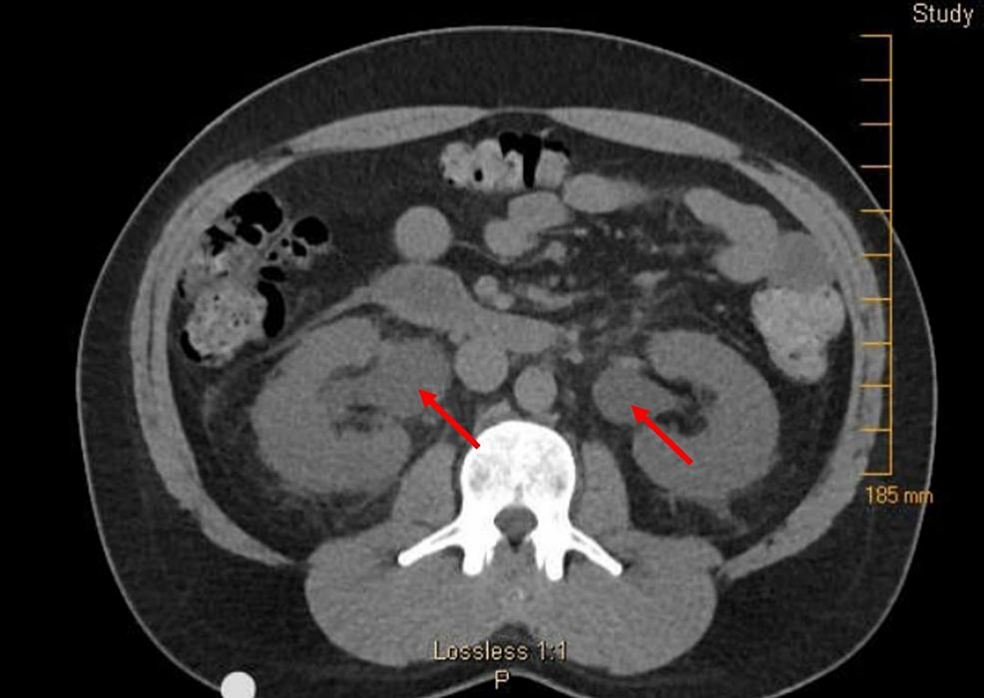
Abdominal and pelvic non-contrast CT revealing bilateral hydroureteronephrosis, suggesting obstruction.
